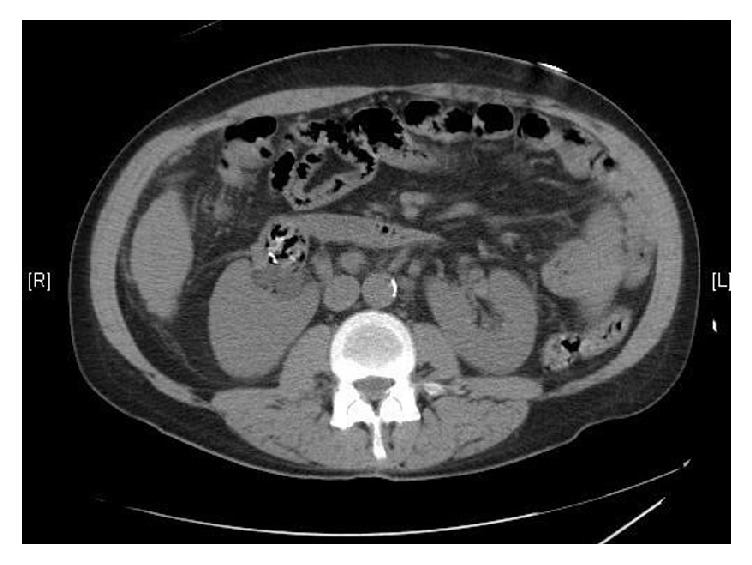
Abdominal CT scan, transverse view. Right renal pelvis collection (with large amount of air and hyperattenuating debris).
